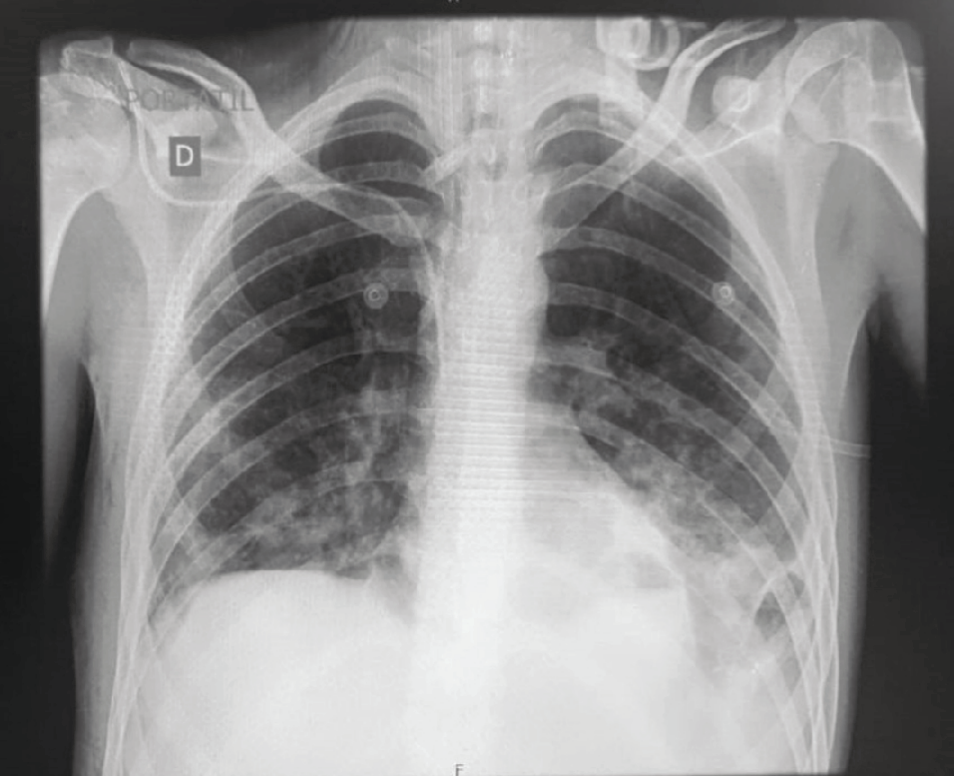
Frontal chest radiograph showing consolidation of both lungs with increasing density towards the lung bases, predominantly the left lung base.
